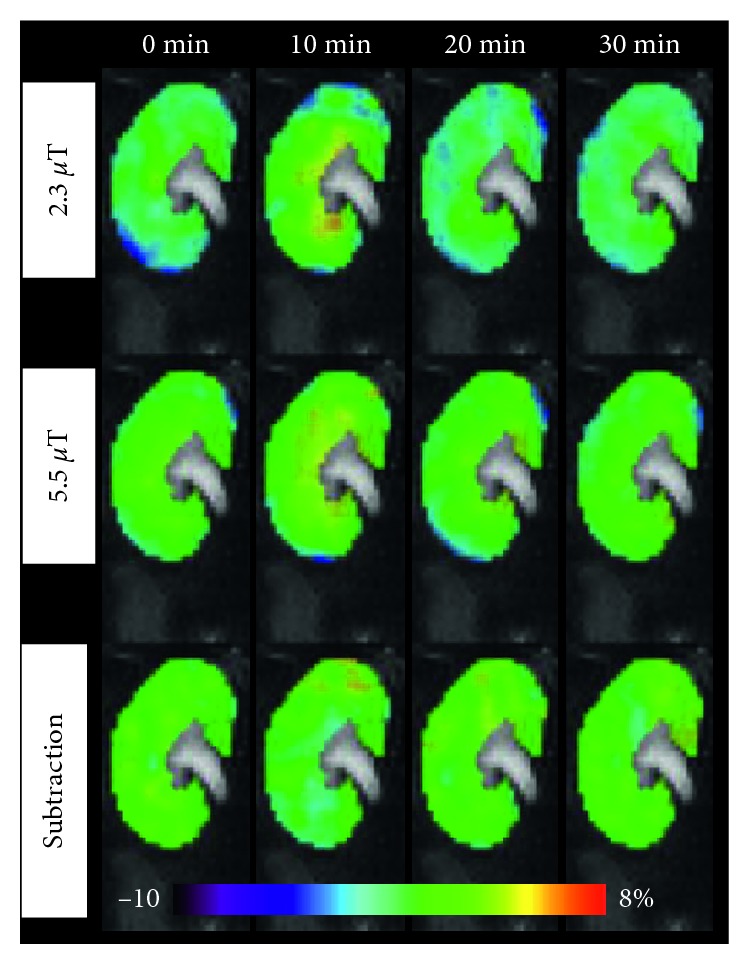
These are a set of representative coronal CEST maps at 3.0 ppm superimposed on T2-weighted images obtained with the presaturation amplitude of 2.3 *µ*T and 5.5 *µ*T, and the subtracted maps between the two power levels before and after injection of the Glu plus high-Nic solutions. The CEST maps are relatively homogeneous over most of the kidney, but small changes are evident after injection of mixed solution, especially in the medulla.
